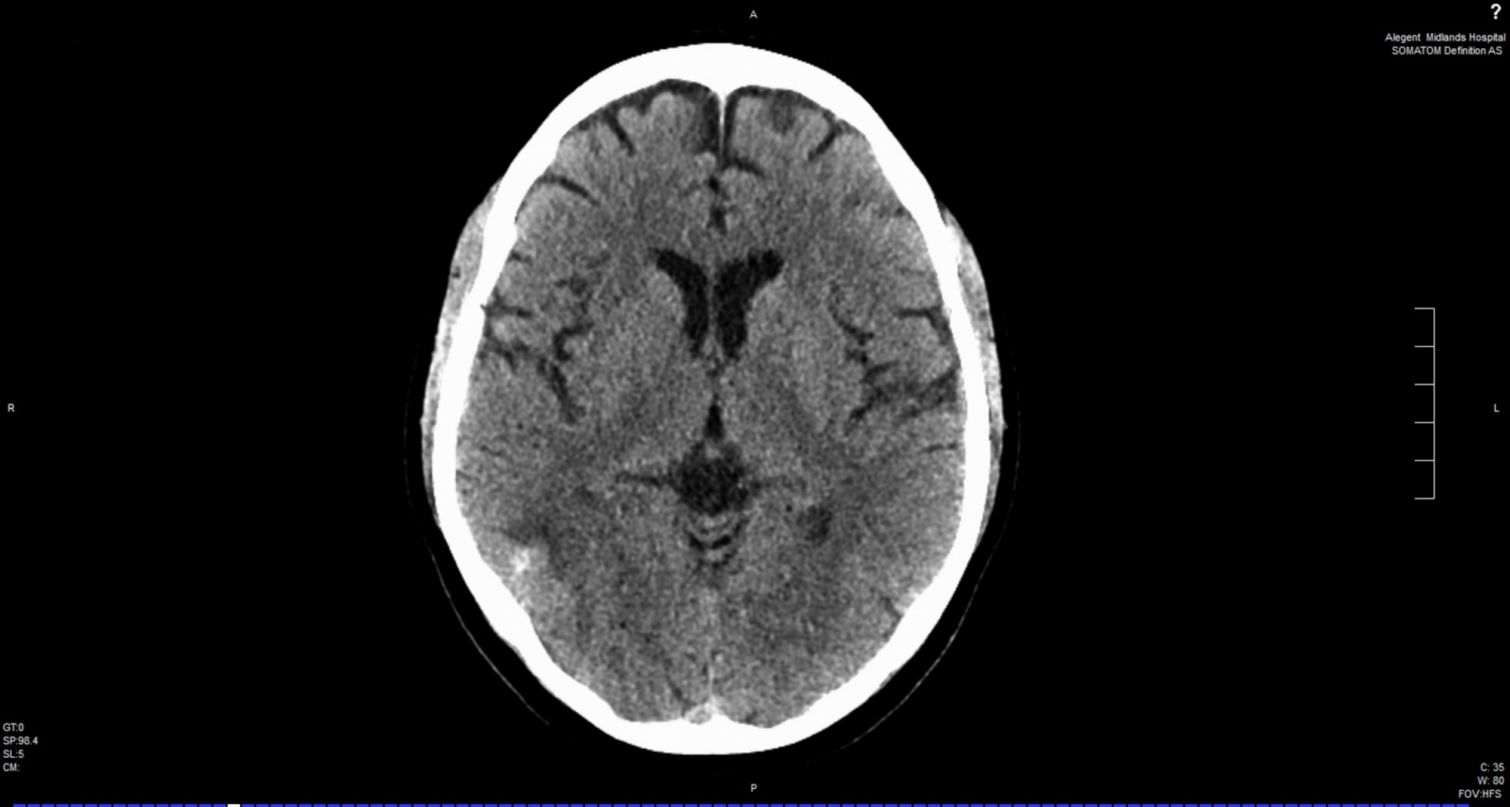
Computed tomography (CT) head without contrast six months later.
